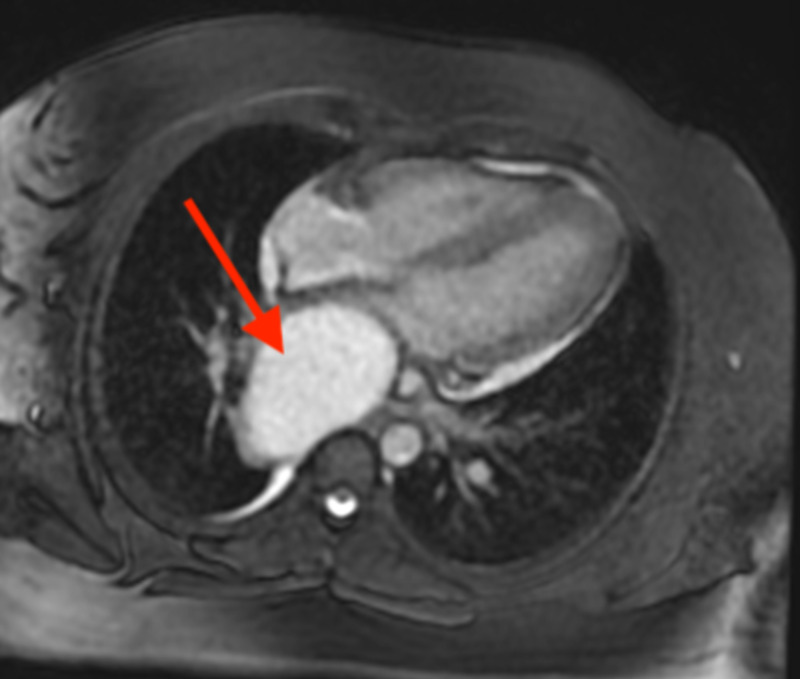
Cardiac MRI (FIESTA, fat-saturated axial four-chamber view) showing uniformly T2 hyperintense retrocardiac mass (red arrow). FIESTA, fast imaging employing steady-state acquisition
